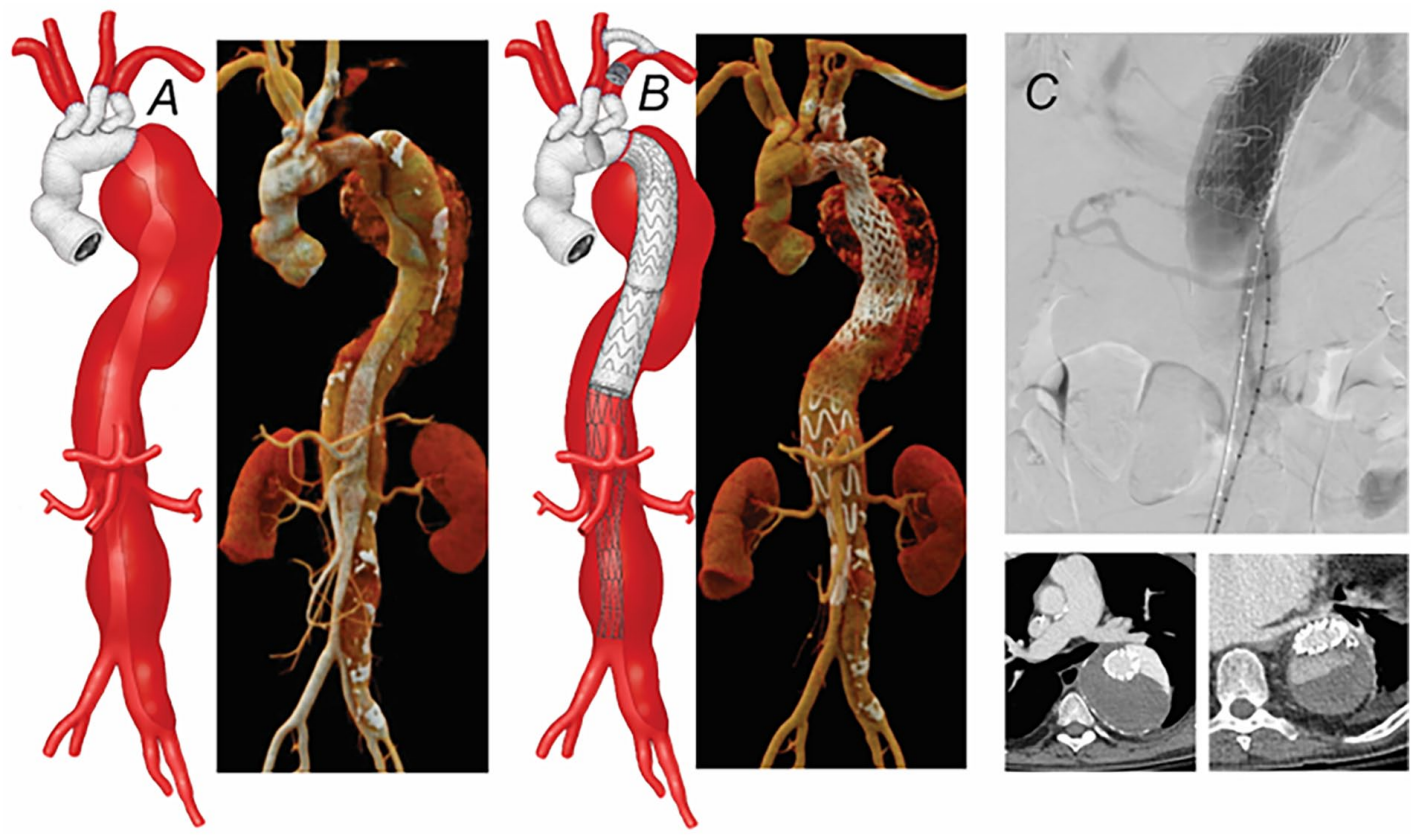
(A) Preoperative 3-dimensional computed tomography angiography (CTA) of 8 cm extent II thoracoabdominal aortic aneurysm after prior total open surgical aortic arch repair using elephant trunk technique. (B) A left carotid-subclavian bypass and thoracic endovascular aortic repair were done as a first-stage procedure. (C) After placement of the thoracic stent-graft to the level of the celiac axis, there was a stent-induced new entry tear from total disruption of the chronic dissection flap, which was treated by PETTICOAT. In-hospital repeat CTA showed a new false lumen with dense contrast opacification.
